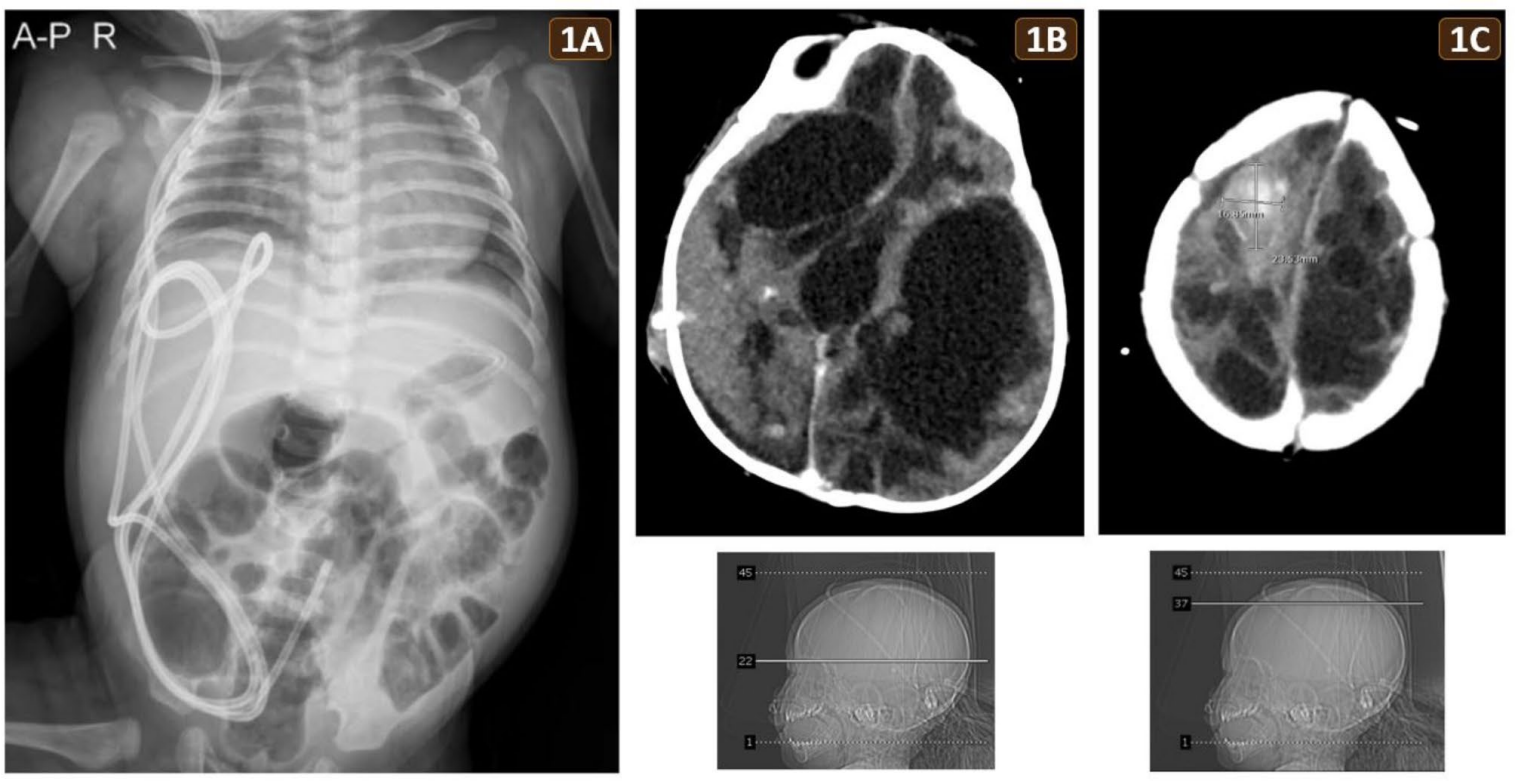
(A) Abdominal X-ray at 2 months of age, taken during an episode of sepsis, showing multiple dilated bowel loops without air-fluid levels or pneumoperitoneum, consistent with marked abdominal distension. The VP shunt was visible. (B, C) Brain CT at 6 months of age revealing extensive bilateral encephalomalacia, hydrocephalus, and a hyperdense lesion (2.4 × 1.7 cm) in the right high frontal lobe, indicative of recent hemorrhage
